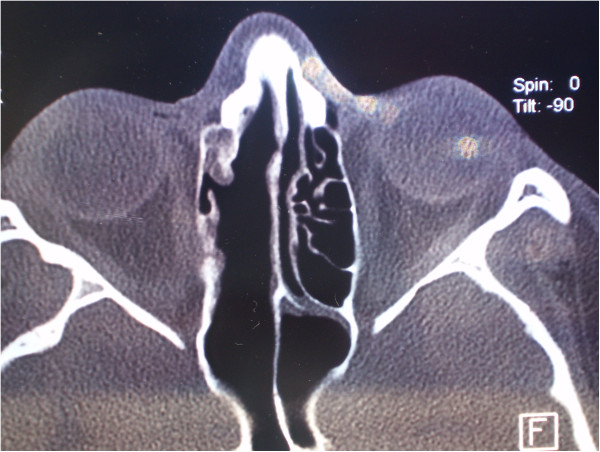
Axial multislice computed tomography one year postoperatively.
